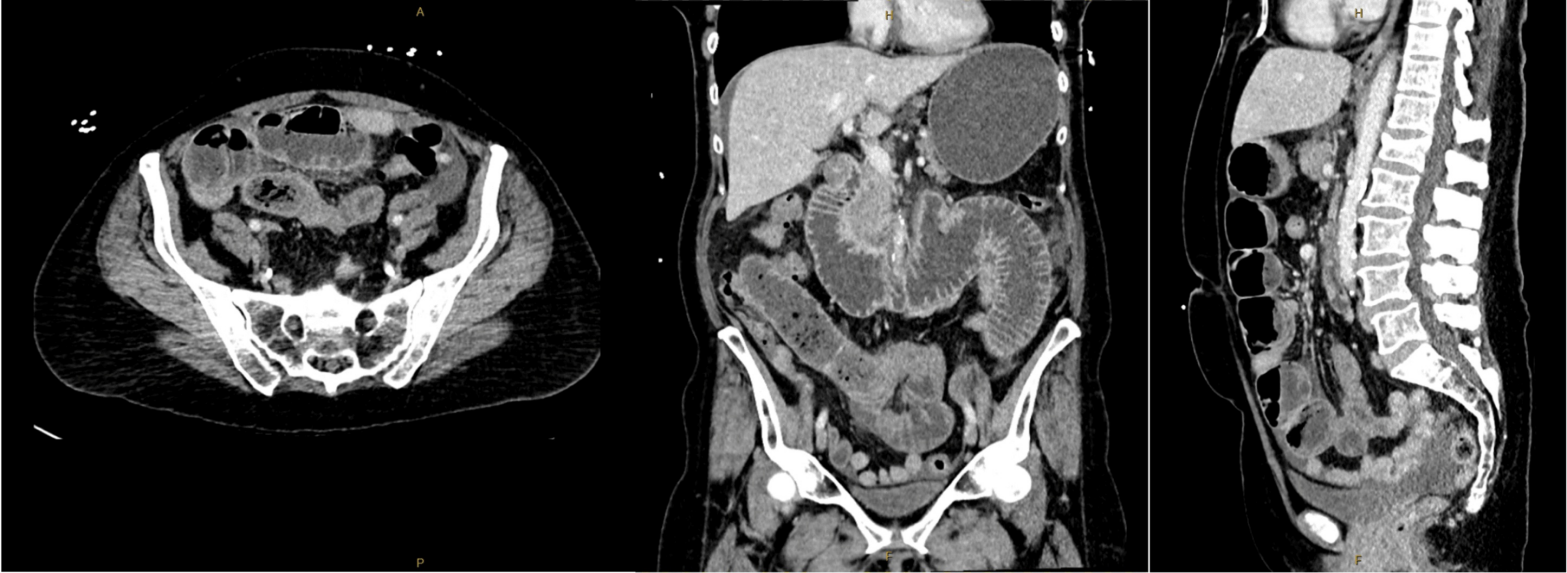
Contrast-enhanced CT scan of the abdomen and pelvis demonstrating dilated small bowel loops with multiple air-fluid levels and a transition point in the low central abdomen. No discrete intraluminal mass or volvulus were identified
